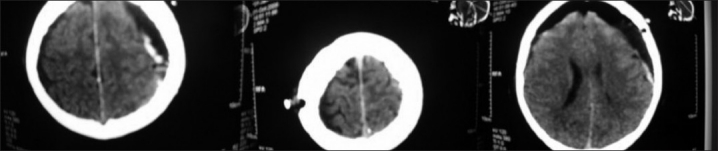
CT brain showing Mount Fuji sign
